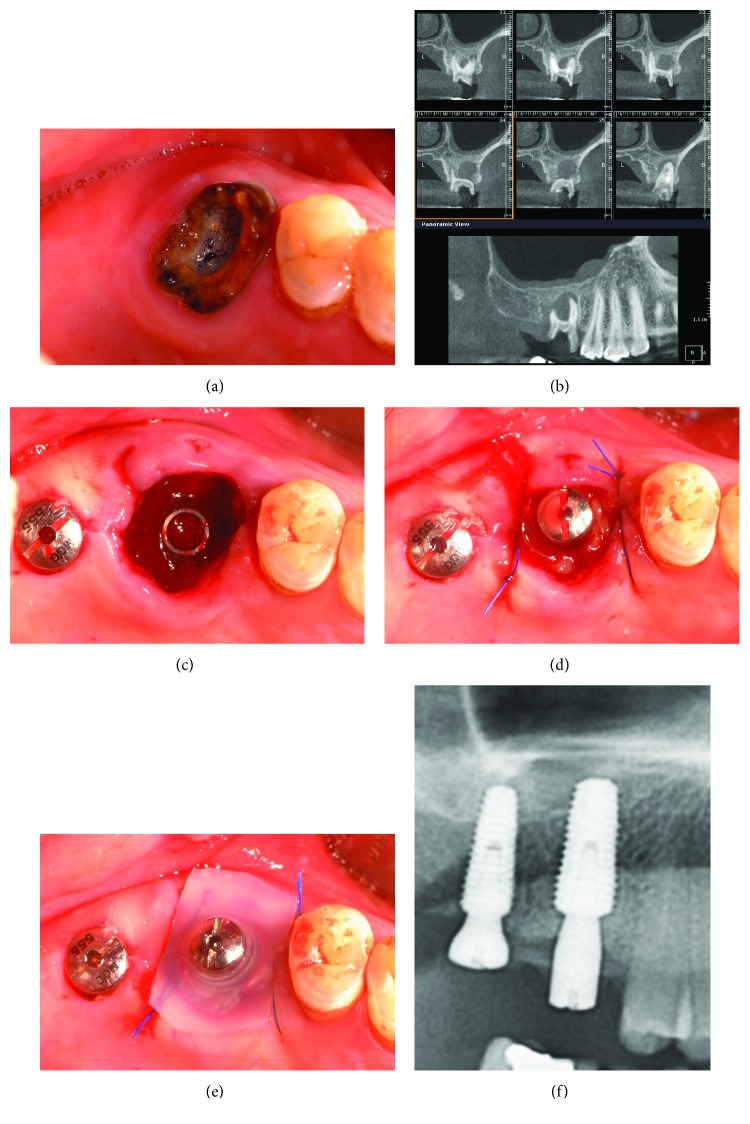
(a) Occlusal view at the initial examination. (b) Cone beam computerized tomography image at the initial examination. (c) Occlusal view immediately after the insertion of the implant. (d) Clinical condition of the interrupted sutures after gap filling of the extraction socket with allogenic bone and PRF. (e) Application of the silicone cap. (f) Radiograph taken immediately after implant insertion.
